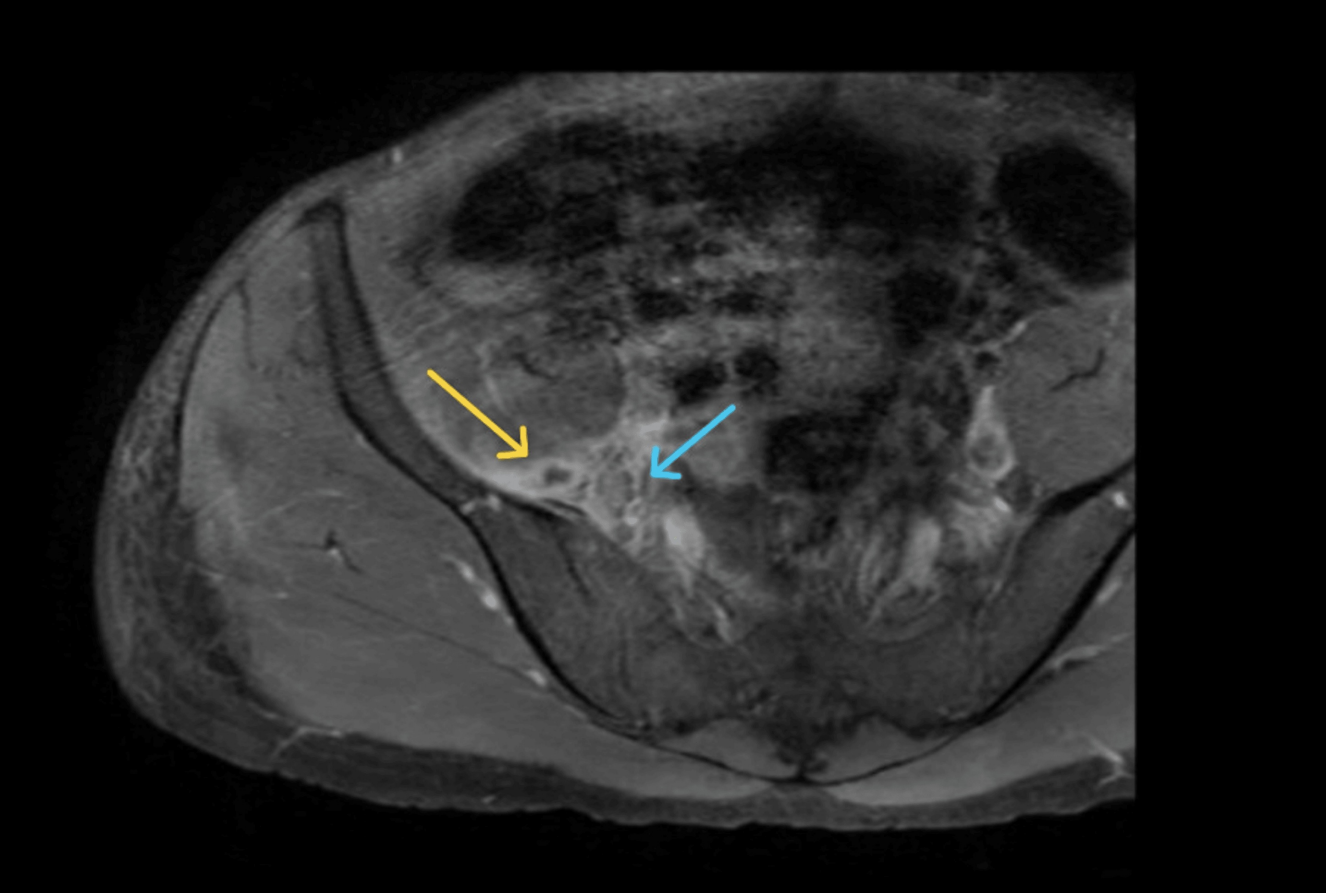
MRI T1-Weighted Image of the Pelvic Region MRI T1-weighted image reveals a small abscess collection (yellow arrow) and an anterior enhancing deep soft tissue adjacent to the para-sacroiliac joint, suggestive of fasciitis (blue arrow). MRI: magnetic resonance imaging.
